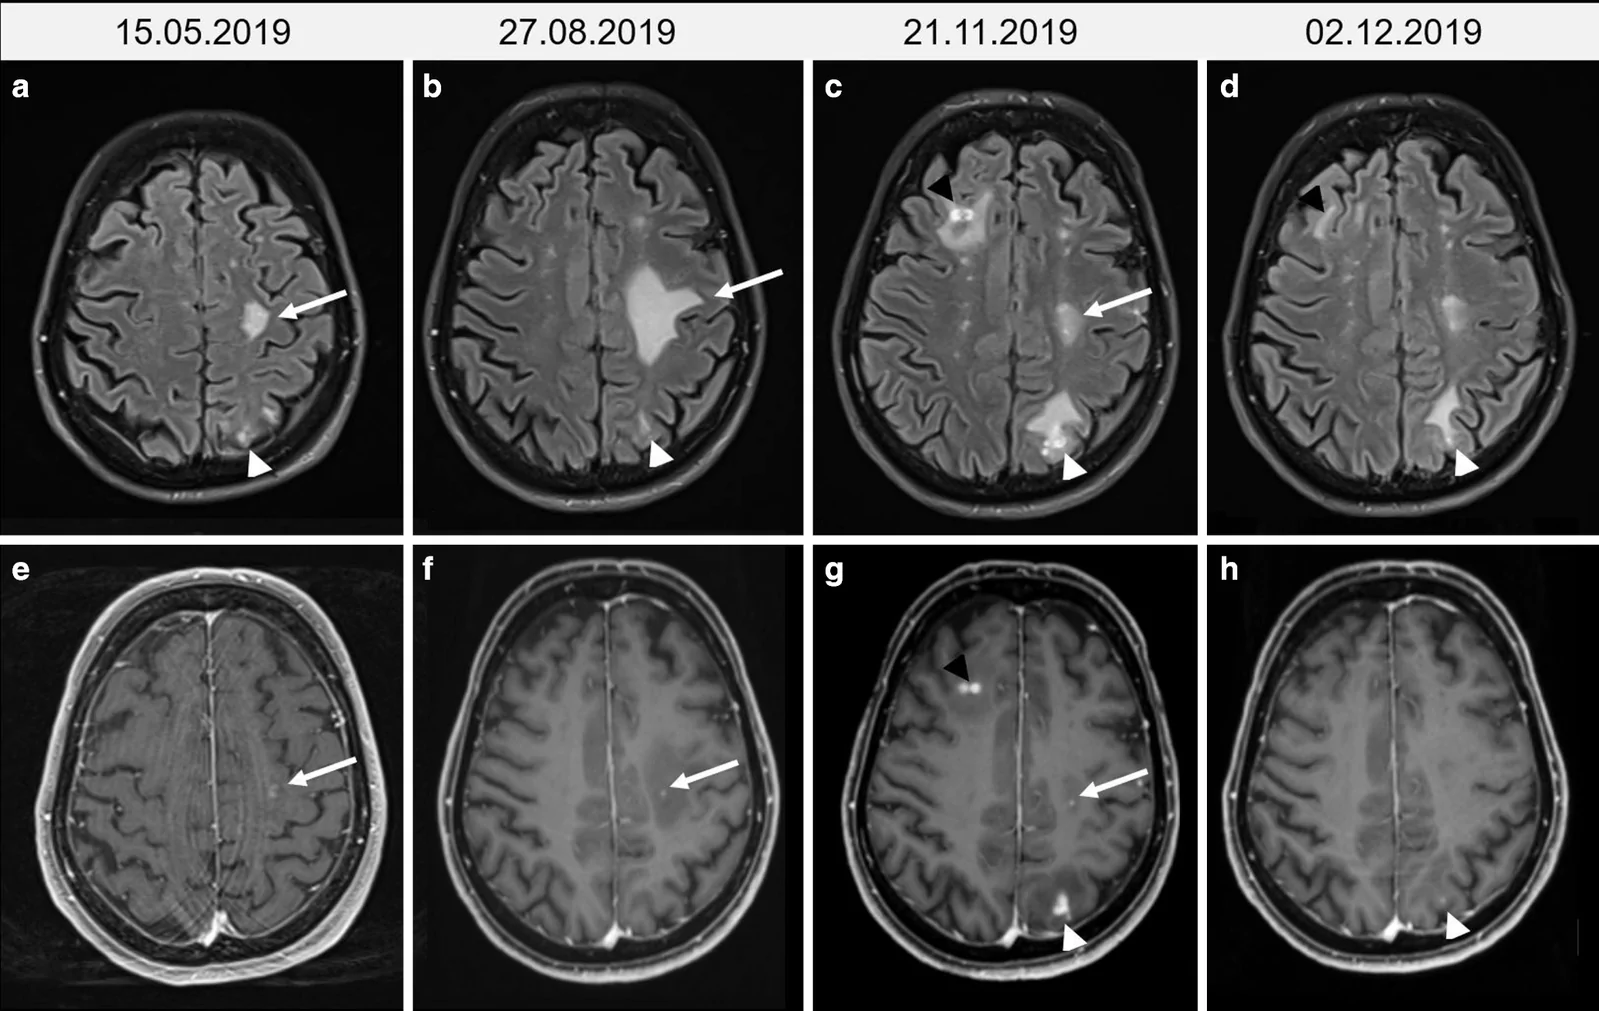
Non ischemic cerebral enhancing (NICE) lesions. a–h initial routine follow-up MRI in a 59-year old woman several months after coil embolization of an anterior communicating artery aneurysm without additional placement of a stent or flow diverter showing several left-sided hyperintense lesions (a arrow, arrowhead; fluid attenuated inversion recovery (FLAIR)), the precentral one with slight enhancement (e arrow); follow-up examination 15 weeks later (b, f) disclosed progressive perifocal signal changes (b, f ; arrow, arrowhead); c, g further deterioration left-sided parietal (c, g white arrowhead) and new right-sided frontal lesions (c, g black arrowhead), regression of the left-sided central lesion (c, g arrow); d, h additional regression of the right-sided frontal and left-sided parietal lesions (d, h white arrowhead; d: black arrowhead). Note that at any time the patient had neurological deficits
